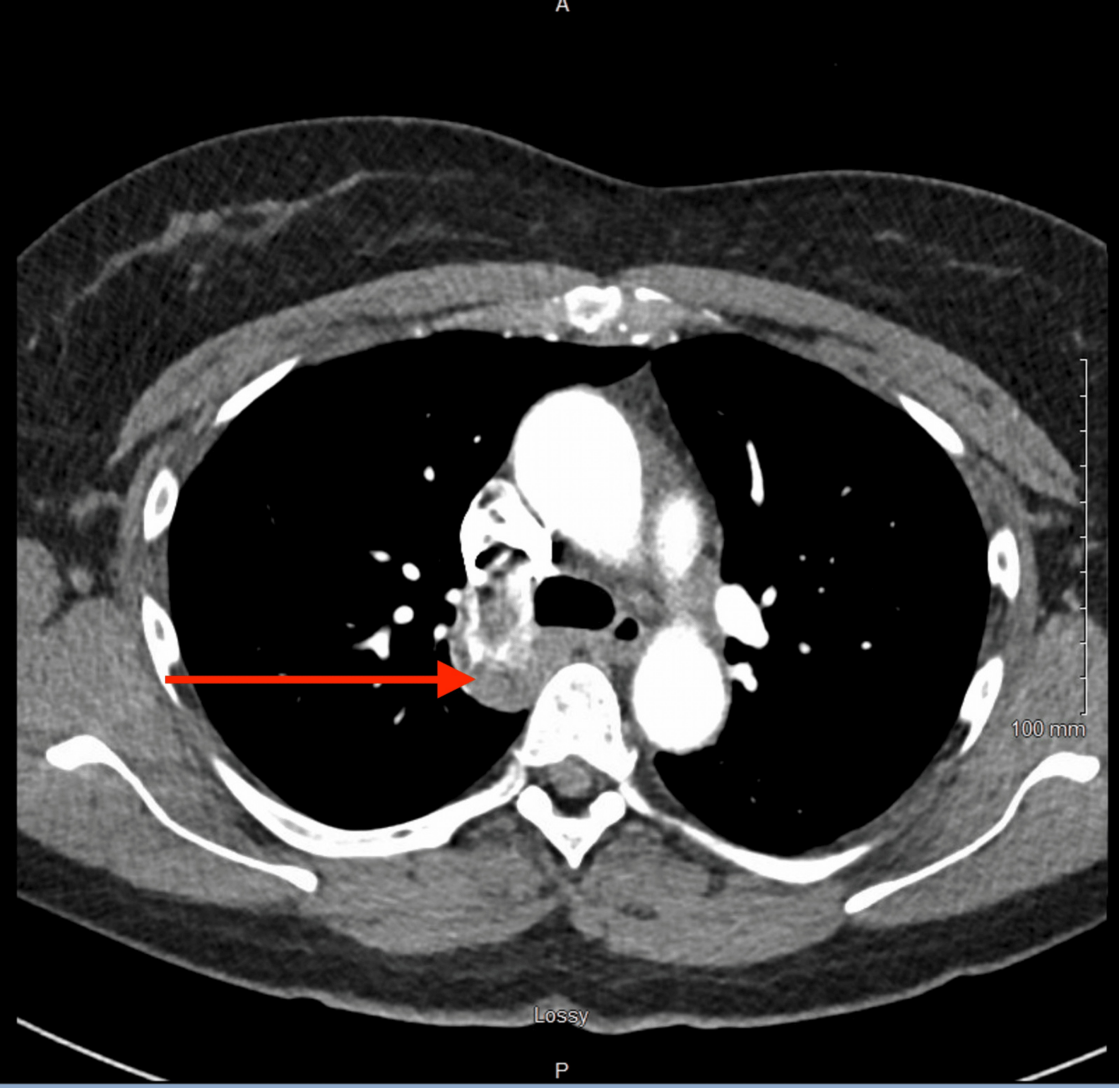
CT (pulmonary embolism protocol) showing an enlarged azygos vein (red arrow) in the thorax
